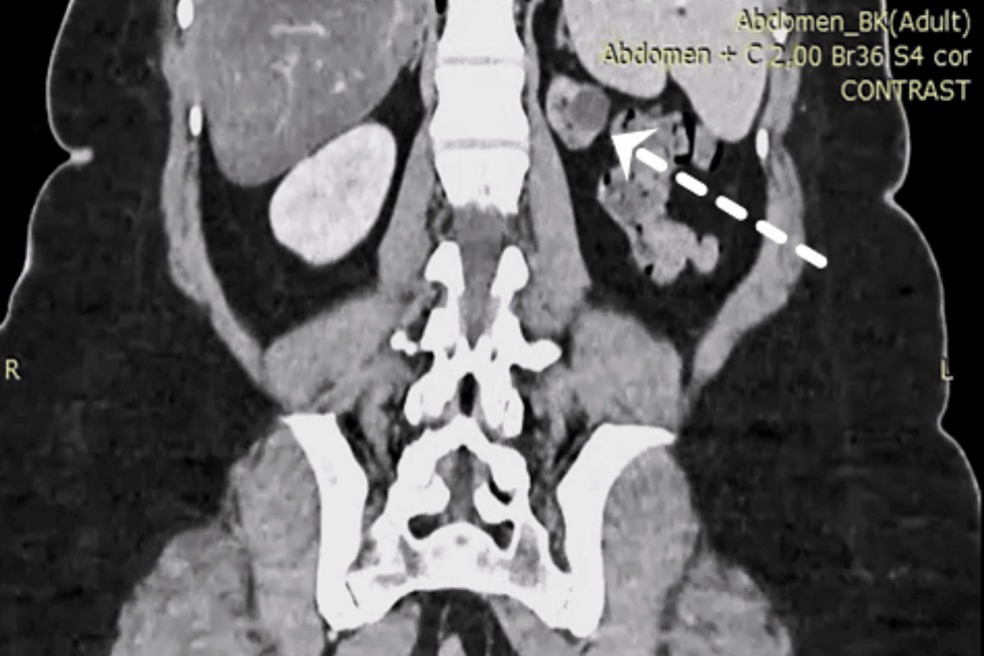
Computed tomography coronal view showing the mass on the left hypoplastic kidney (arrow)
